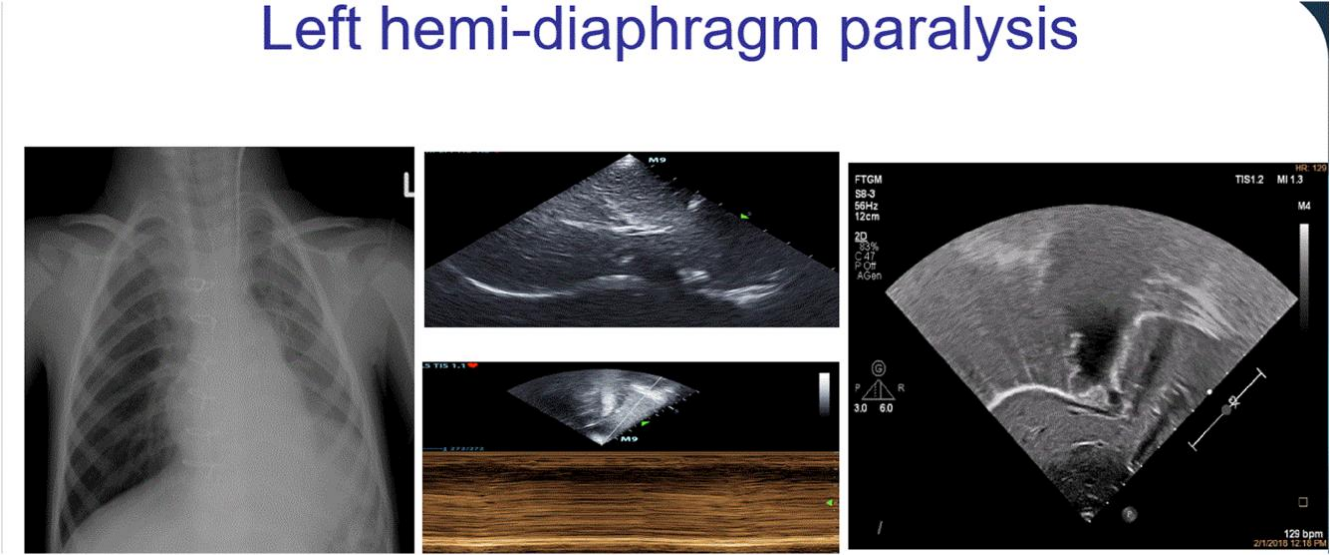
A left hemidiaphragm paralysis can be visualized by chest X-ray (left side) and confirmed by echographic analysis of the diaphragm by subcostal view. In the middle, a paradoxical motion of the diaphragm can be appreciated in M-mode, and on the right image, the left hemidiaphragm lifted can be appreciated (Supplementary data online, *Video S2*).
